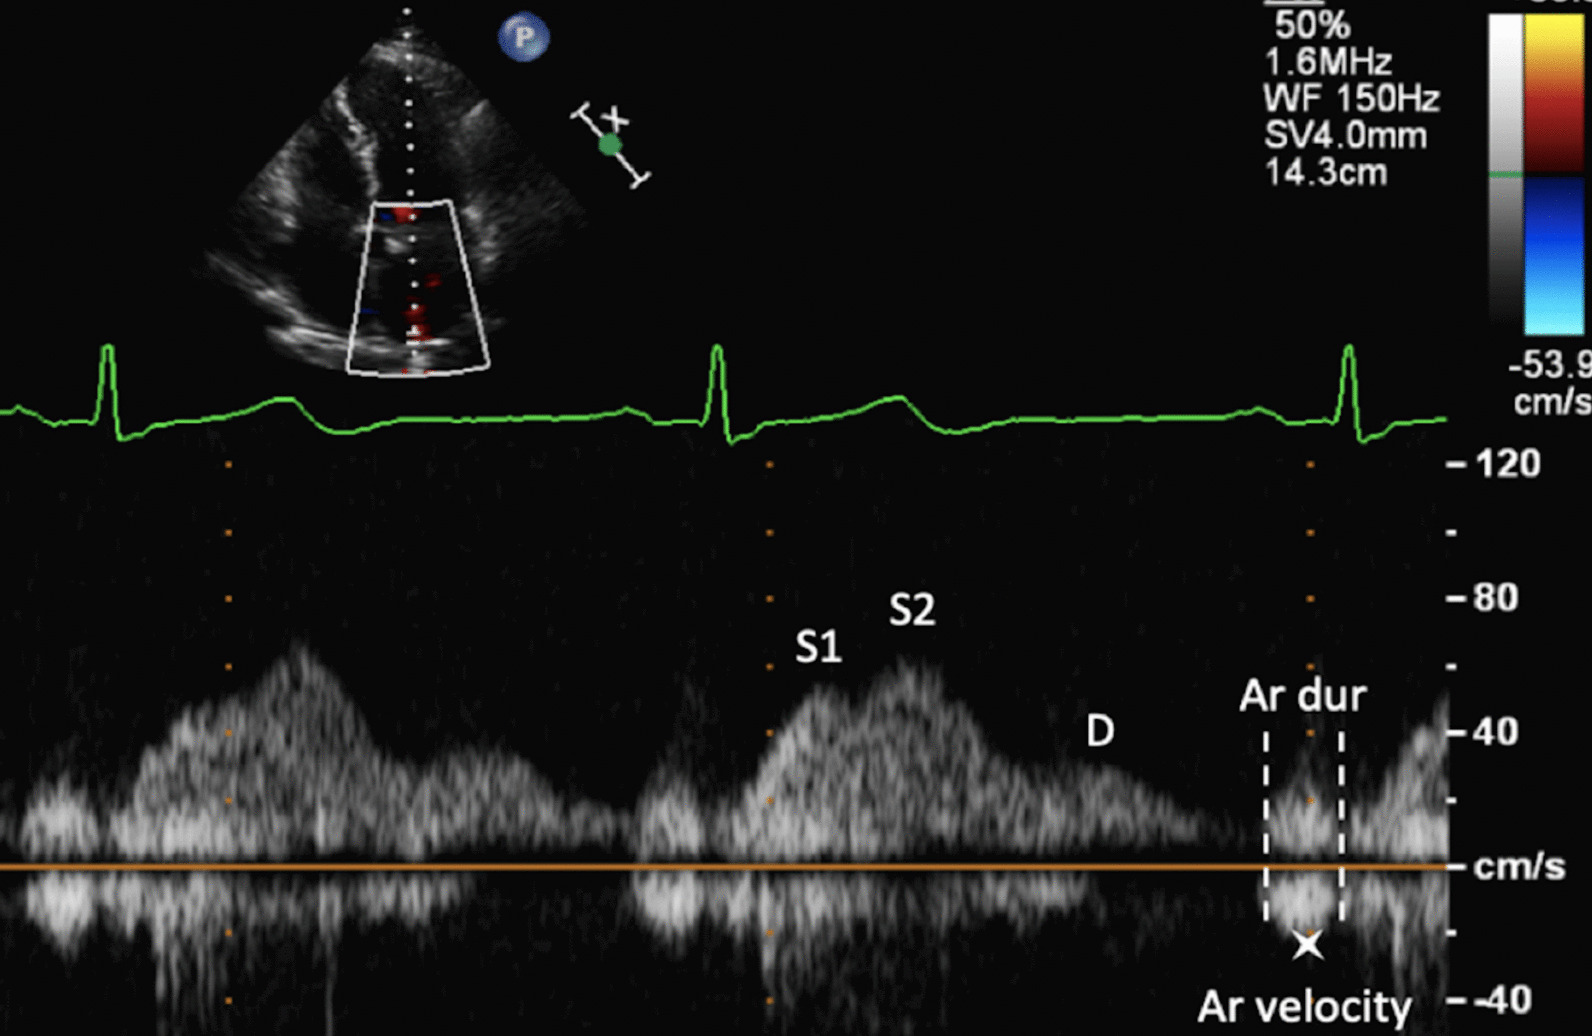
PV Doppler: S^1^ and S^2^ waves occur in systole, contributed to by elastic recoil of the LA, LV systolic shortening and RV SV/SPAP propagating through the lungs. The D waves occurs with LV relaxation in early diastole while the Ar wave occurs in late diastole following atrial contraction
